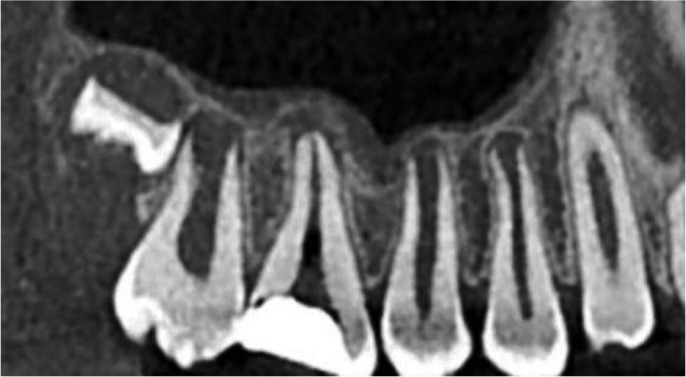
Preoperative radiographic view of maxillary first molar.
